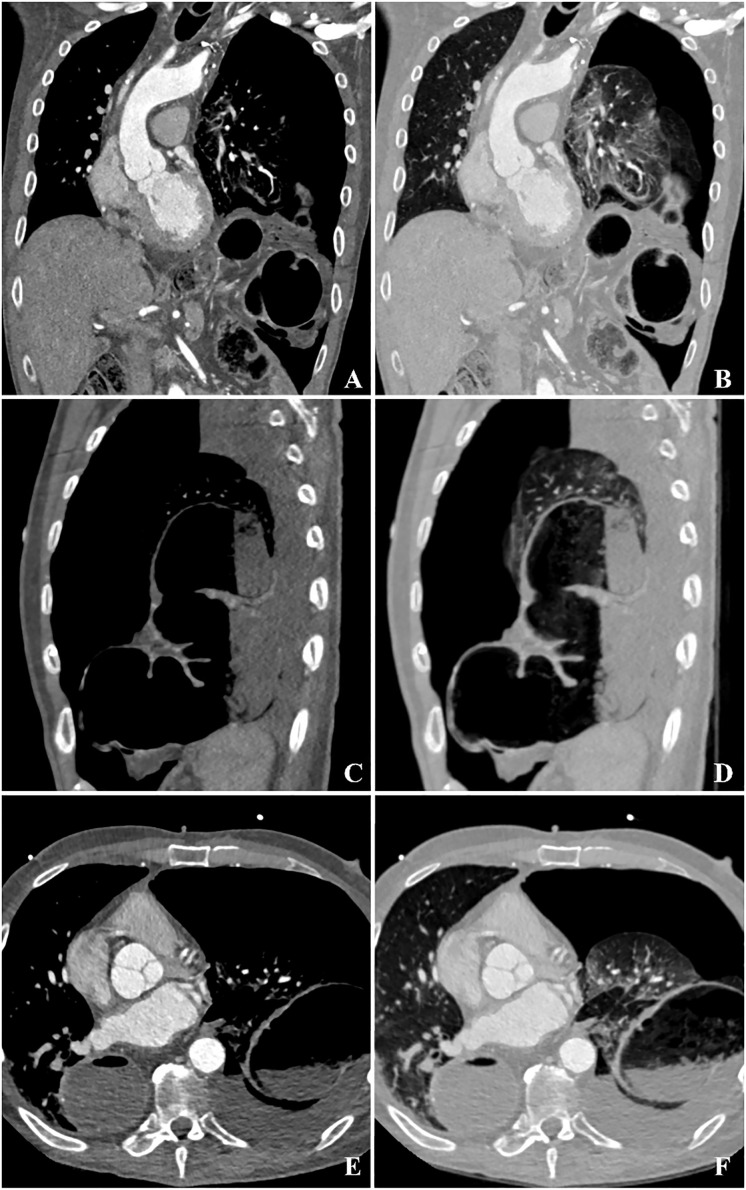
Triple-rule-out CT imaging of the described patient in coronal (A, B), sagittal (C, D), and transverse (E, F) reconstructions. (A, B) show a left-sided tension pneumothorax with mediastinal displacement to the left. In the hiatal area, torsion of the transverse colon and mesocolon reveals a closed-loop obstruction. A density-increased pleural effusion and dilated transverse colon in the left hemithorax can be seen (C-F), suggesting a colonic perforation as cause for pneumothorax.
